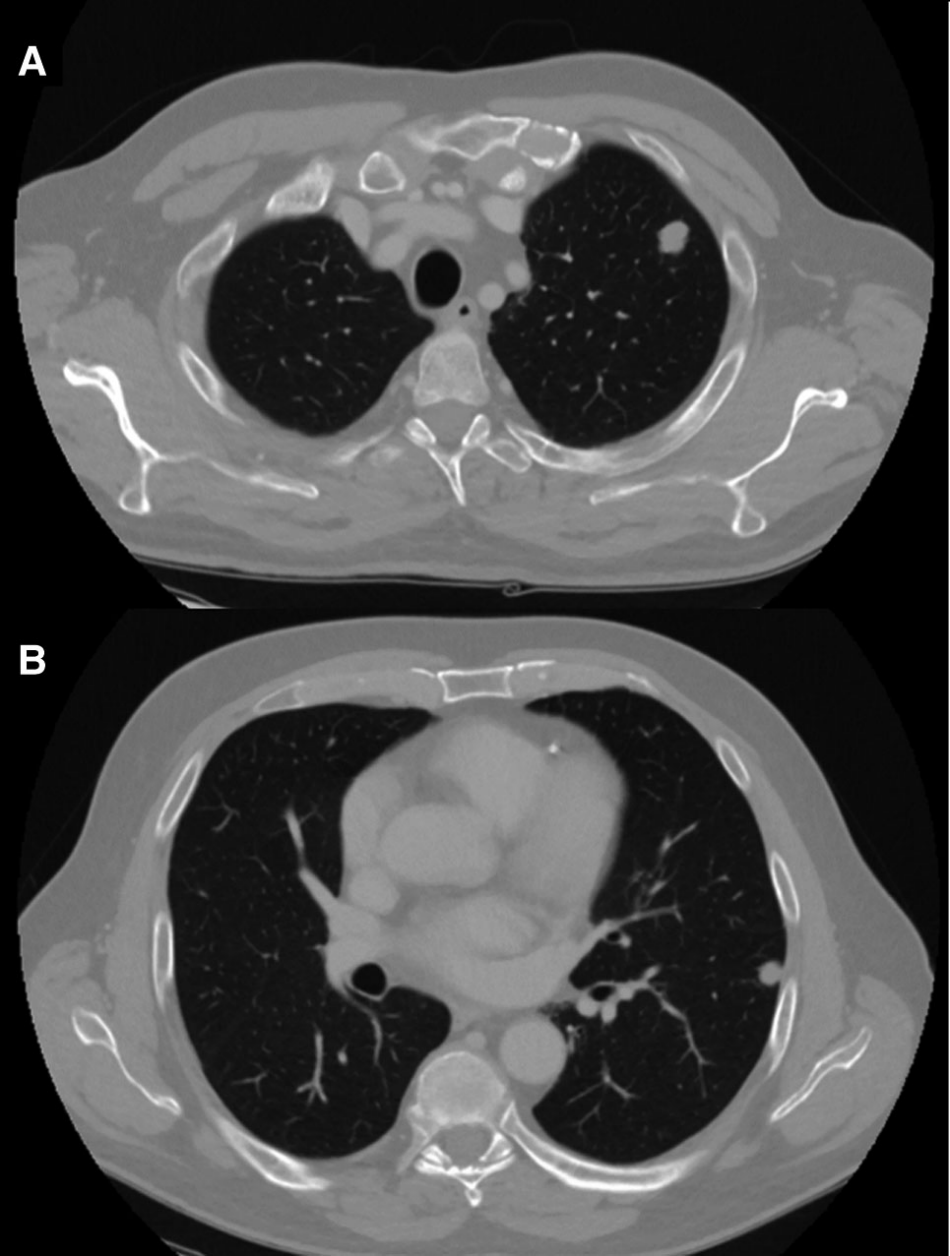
CT scan of two left lung nodules: (A) 15 mm upper lobe; and (B) 12 mm lower lobe.
